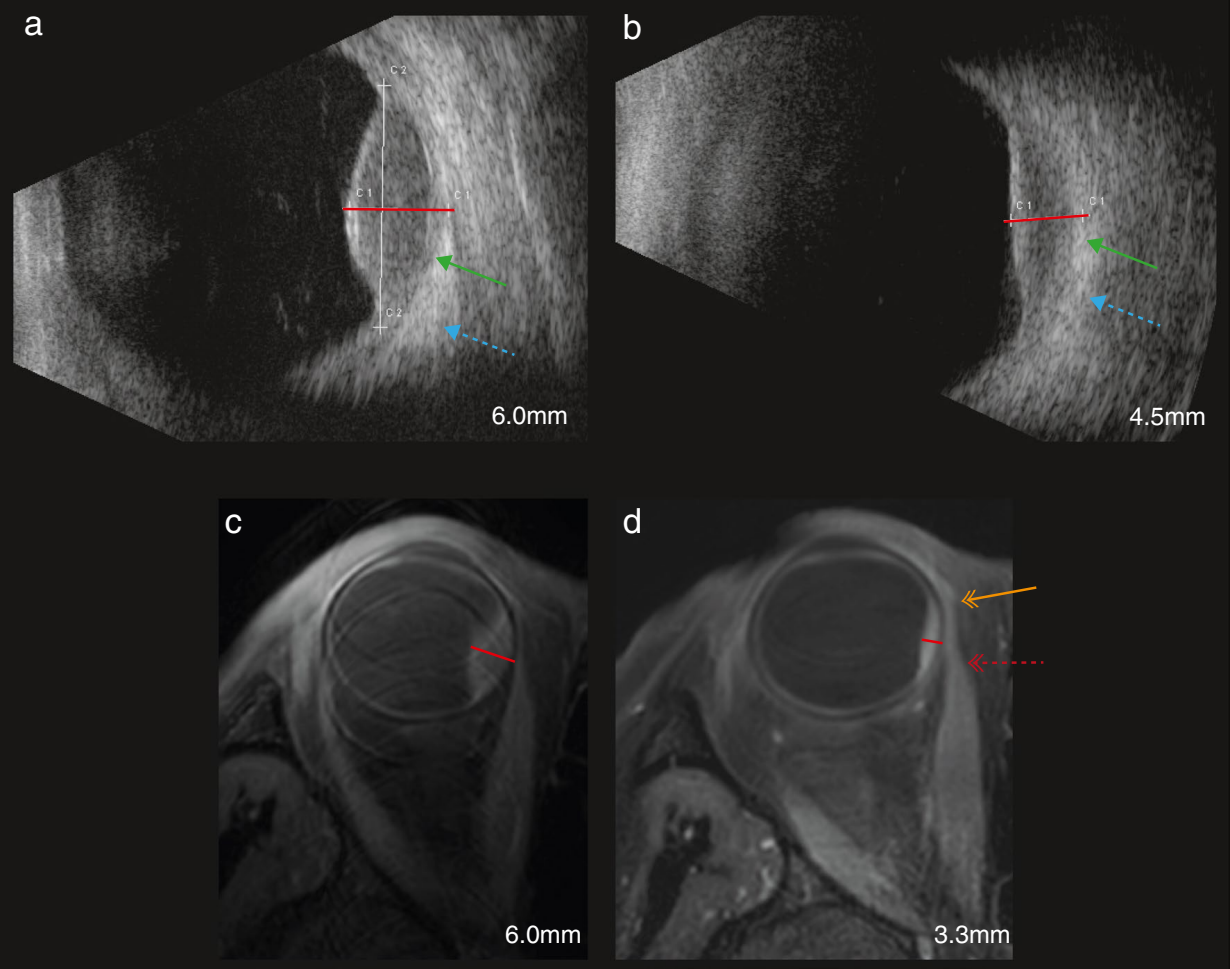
a, b Ultrasound and c, d MRI of a UM of the right eye a, c before and b, d 3 months after ruthenium plaque brachytherapy. Post-treatment changes, accounting for less accurate ultrasound measurements of the UM after radiotherapy, but not interfering with the MR measurements. The tumour prominence measured 1.2 mm thicker on ultrasound as compared to MRI (red lines) after brachytherapy, which could likely be attributed to structural changes of the extra-ocular tissue due to radiation reactions or due to the temporary surgical detachment of the extra-ocular muscle adjacent to the tumour needed to facilitate the placement of the ruthenium applicator. a, b Ultrasound images with the identification of the limits of the UM, of the sclera (green arrow) and of the medial rectus (blue dashed arrow) being easy before and difficult after radiotherapy. c, d Axial oblique MS TSE contrast-enhanced T1 with fat signal suppression. Although there is post-radiotherapy peri-scleral enhancement (orange arrow with double chevron), and thickening of the tendon of the medial rectus due to its intraoperative detachment during the ruthenium plaque insertion (red dashed arrow with double chevron), the limits of the tumour and of the sclera remain clearly visible, allowing accurate measurements
